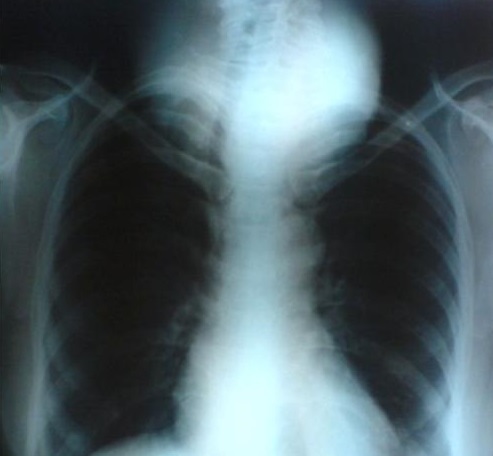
Chest X-ray: the right eccentricity of the trachea
